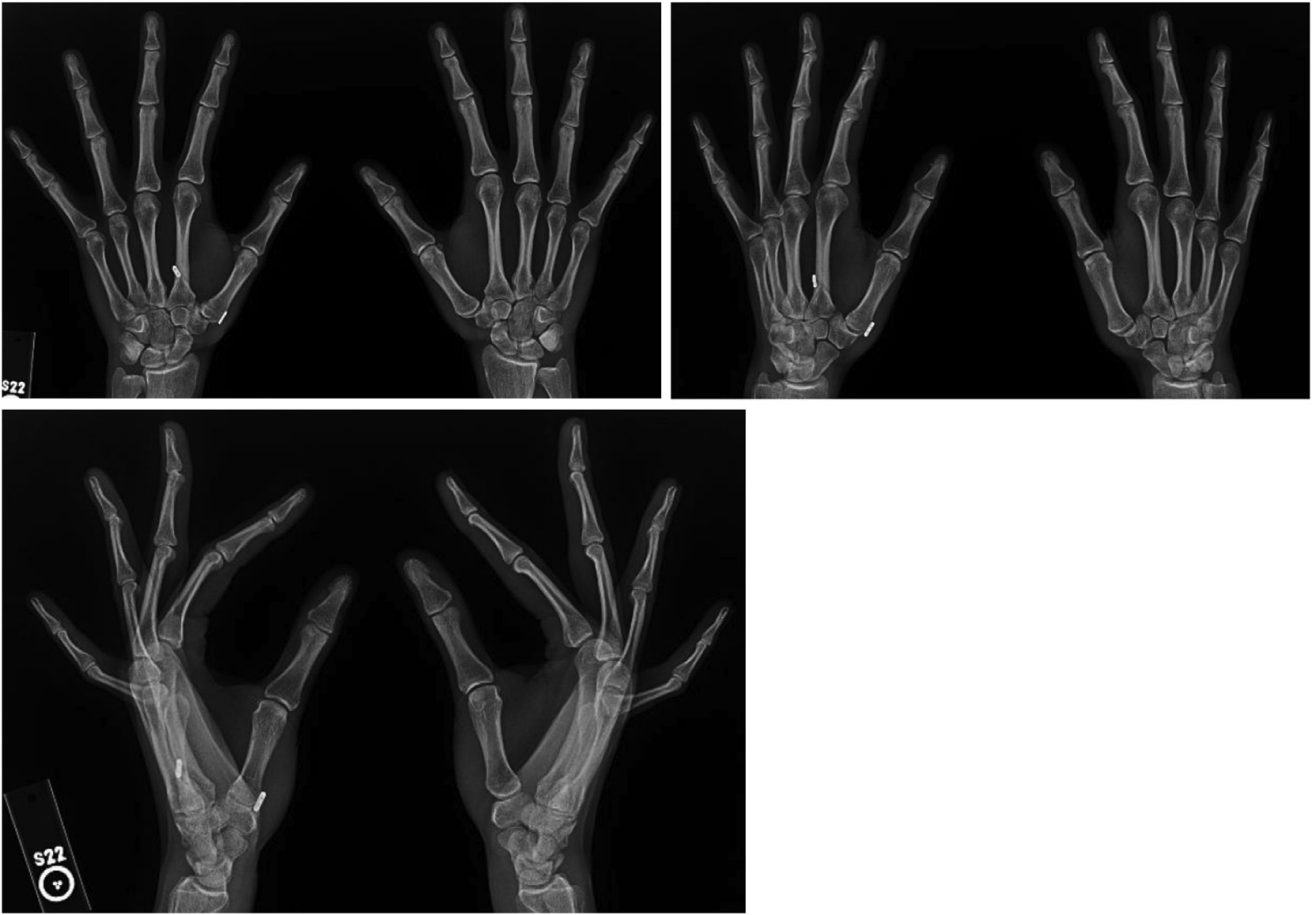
Fifteen-month postoperative posteroanterior, oblique and lateral bilateral hand radiographs with improved left thumb CMC alignment.
